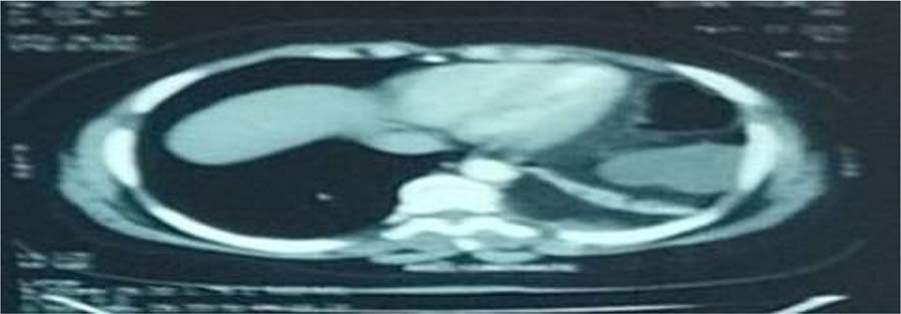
Axial section of an abdominal-pelvic CT scan showing a strangulated Bochdalek hernia with colonic and omental contents.
